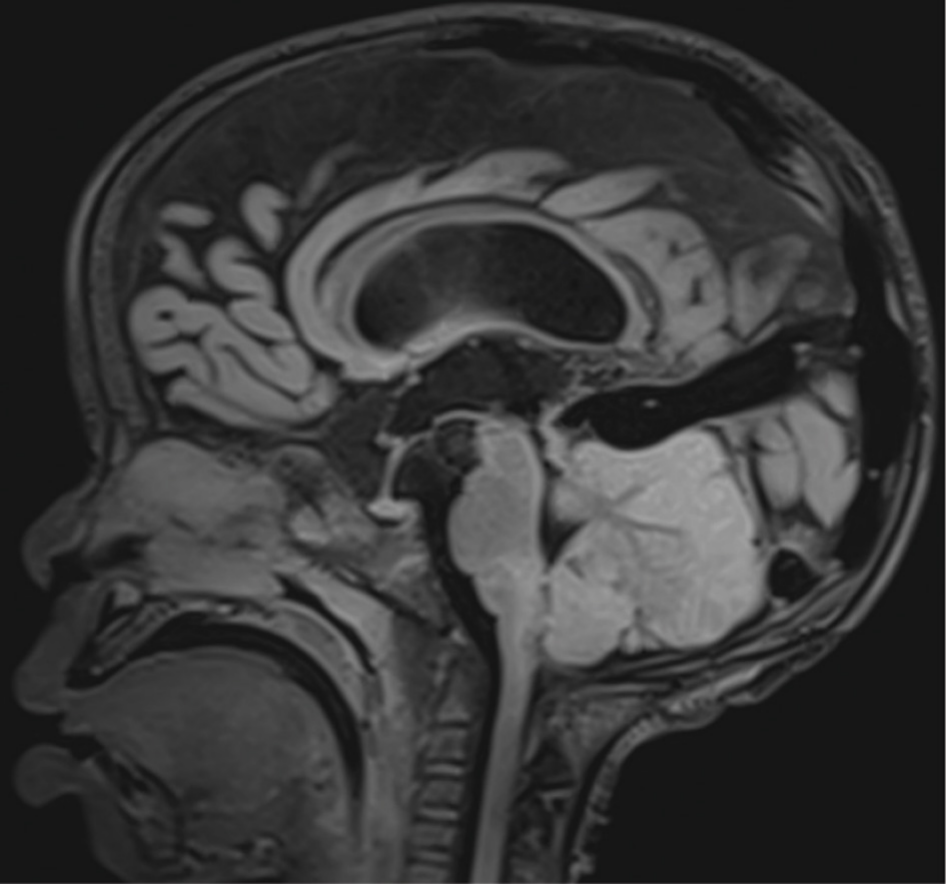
Sagittal T2‑weighted MRI showing an intense signal in the cerebellum, causing mass effect with anterior displacement of the medullar bulb.
